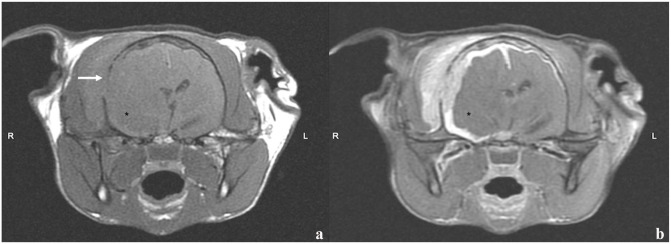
Transverse T1-weighted (T1W) (a) pre- and (b) postcontrast images of the brain. There is diffuse cerebral grey matter swelling along the right cerebral convexity and severe regional to diffuse pachy- and leptomeningeal thickening with severe, mildly heterogeneous, contrast enhancement. The intracranial lesion extends extracranially into a right temporal muscle lesion through an osteolytic defect in the right temporal bone (arrow). The poorly marginated space-occupying lesion (*) at the level of the piriform lobe is T1W iso- to mildly hypointense and not contrast-enhancing
